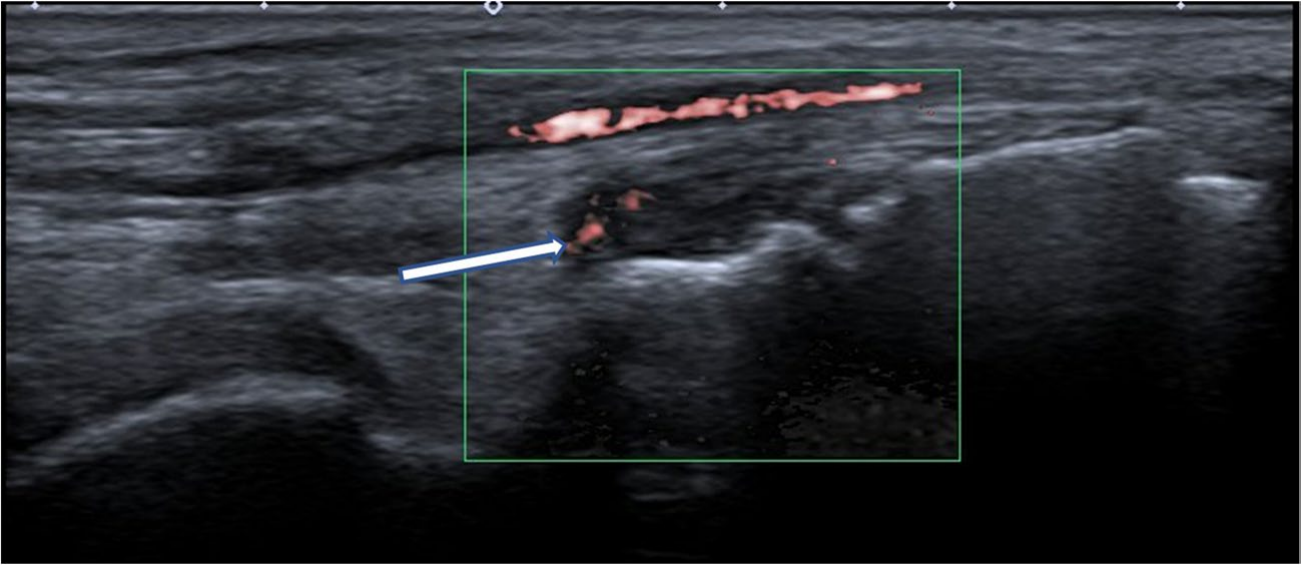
Power Doppler ultrasound of left talonavicular joint in sagittal projection in a 19-year-old young woman with a long history of aggressive seronegative polyarticular juvenile idiopathic arthritis, onset at the age of 7 years and with poor adherence to treatment (same patient as in Fig. 3) shows thickened and chronically altered synovium without a joint effusion or significant hyperemia (*arrow*)
